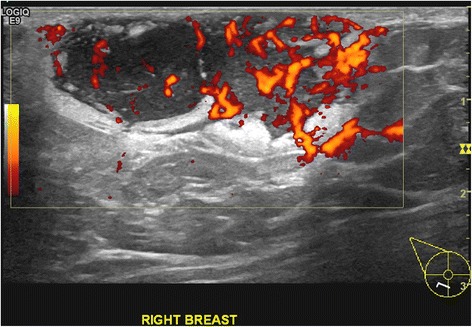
Ultrasound with power Doppler showed high vascularity of the mass.
